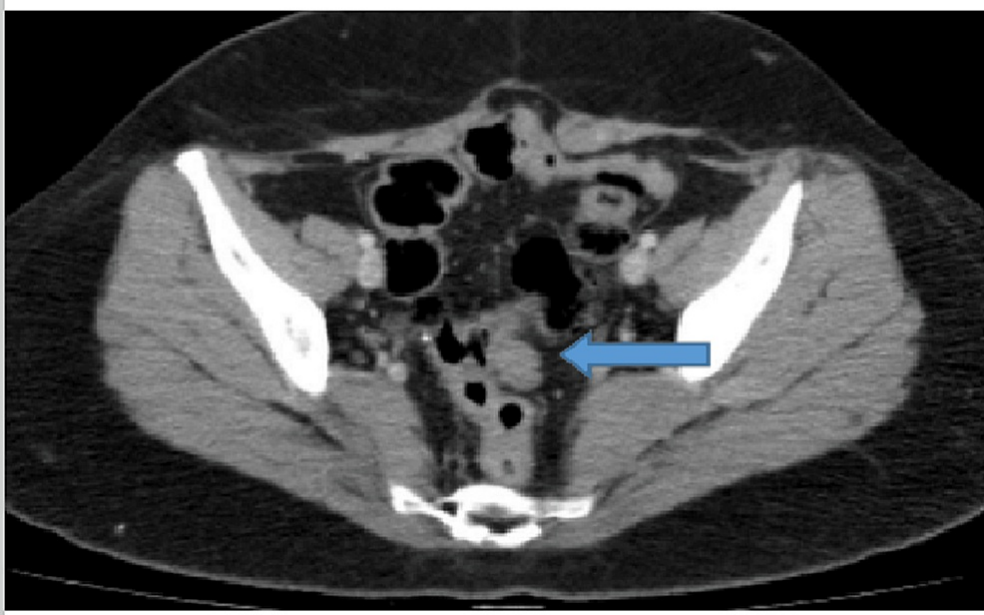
Axial view of CT of the chest, abdomen, and pelvis (CAP) with contrast showing an increase in the size of soft tissue adjacent to the sigmoid colon with an impression of follicles, representing residual ovarian tissue.
